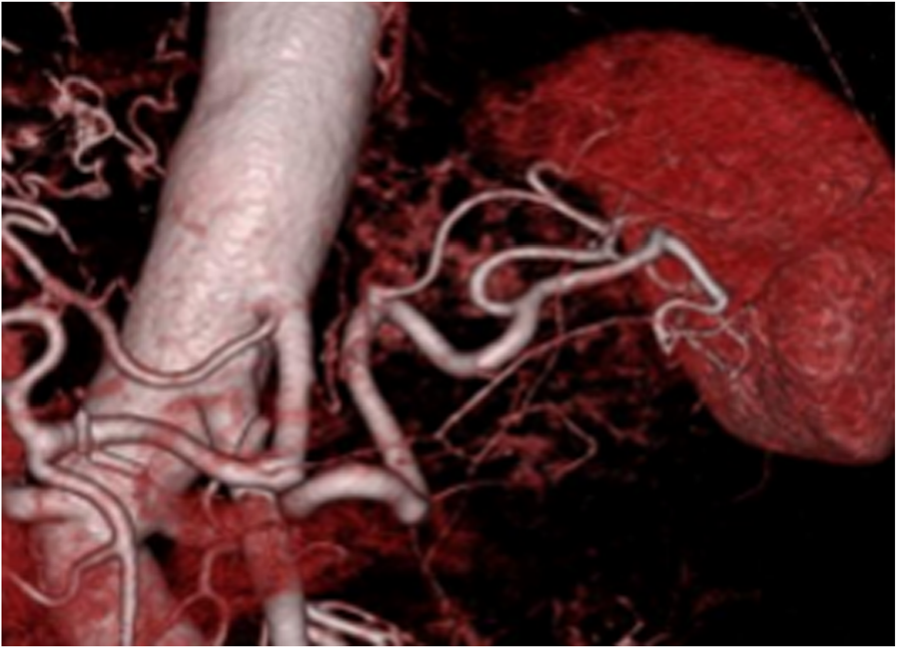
3D-CT angiography to evaluate the formation of the vessels at the hilum of the spleen
